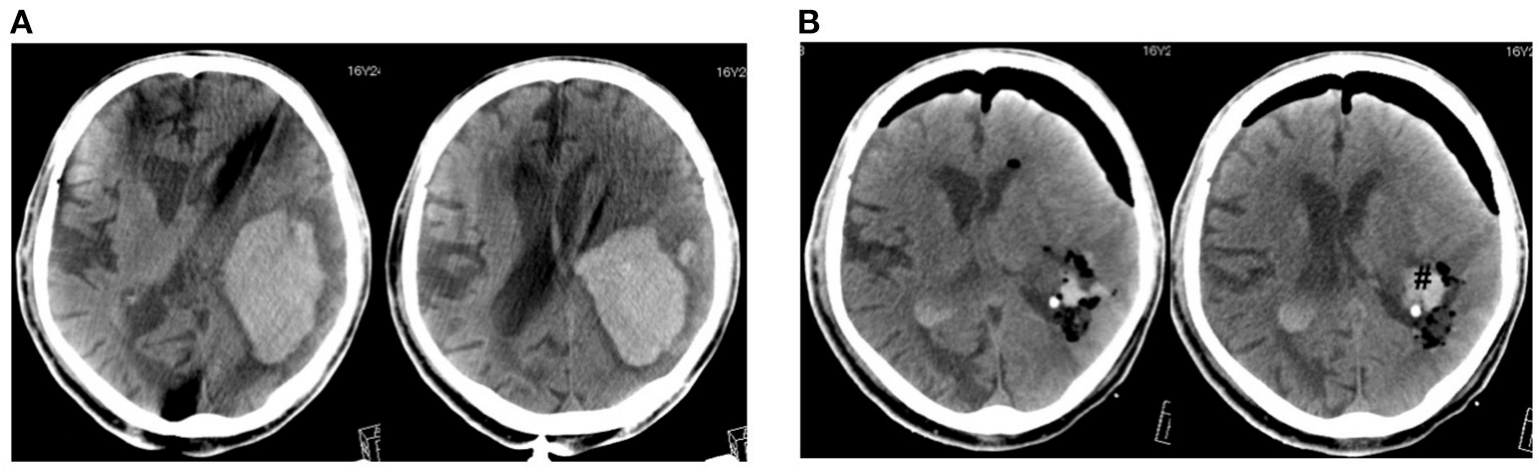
A case of large lobar hemorrhage (110 ml). CT scans before (A) and after operation (B). ^#^Hematoma remnant.
